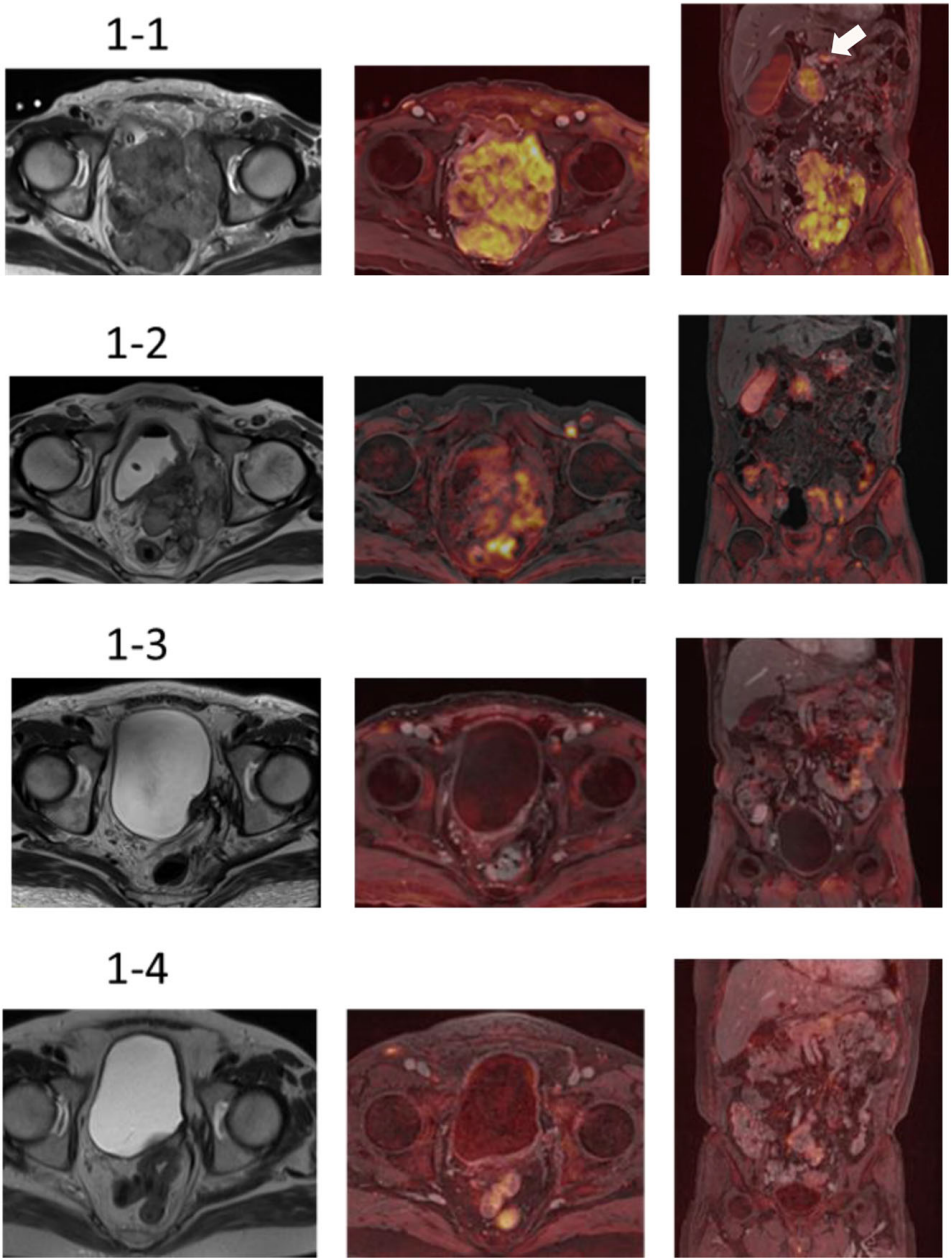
(1‐1) Initial MRI reveals an irregular mass occupying the pelvis, which appears to be primary prostate cancer. The image shows initial evidence of invasion into the surrounding tissues and multiple distant metastases. Arrows indicate pancreatic metastases. (1‐2) MRI after one course of treatment reveals a prominent reduction in tumor size and a trend toward improvement in invasion into the surrounding tissues. (1‐3) MRI after five courses reveals that most of the initial imaging findings have disappeared, including the primary tumor and distant metastases. (1‐4) MRI 1 year after the initial diagnosis. The initial imaging findings are absent.
